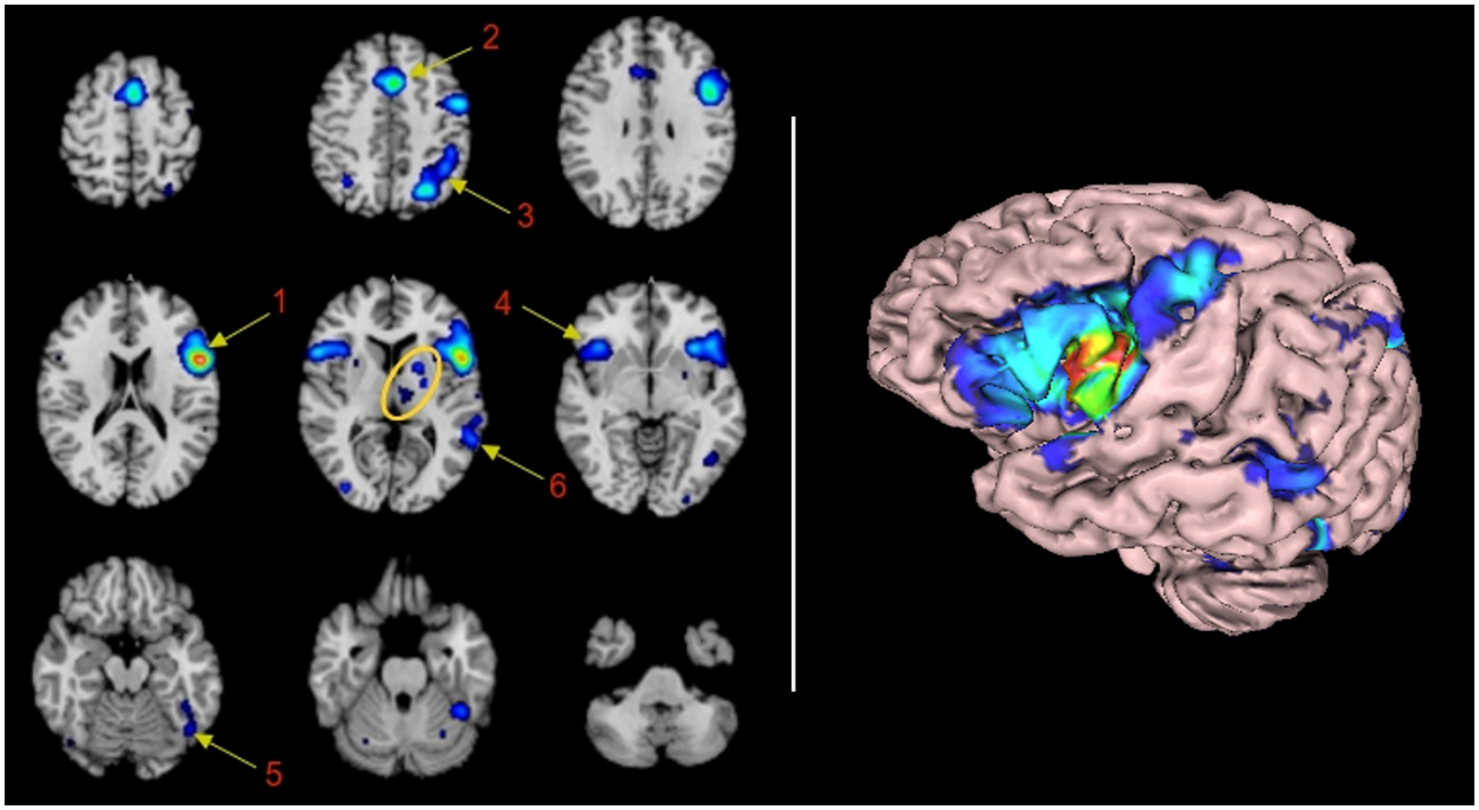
Functional connectivity map of BA44 by Meta-analytic connectivity modeling. (Left) Transversal descending cuts of the brain MRI template. Left hemisphere appears on the right side (Radiological convention). Clusters of activation are color coded for statistical significance from dark blue (lowest) to red (highest). Cluster numbers of the automatic activation likelihood estimate (ALE) report are associated with the main clusters of image. Arrows point approximately to their isocenters. Within the yellow oval, cluster 7 corresponds to the left thalamus, with medial localization, and cluster 8, lenticular nucleus, with lateral and rostral position. The cerebellar activation shown in the middle inset of the lower row is part of cluster 5. It is most likely explained by the smoothing effect of the adjacent activation of the left fusiform gyrus. (Right) 3D volumetric rendition of the brain showing activation on the left hemisphere surface. Red color zone identifies BA44. Deep and midline activations are not shown.
